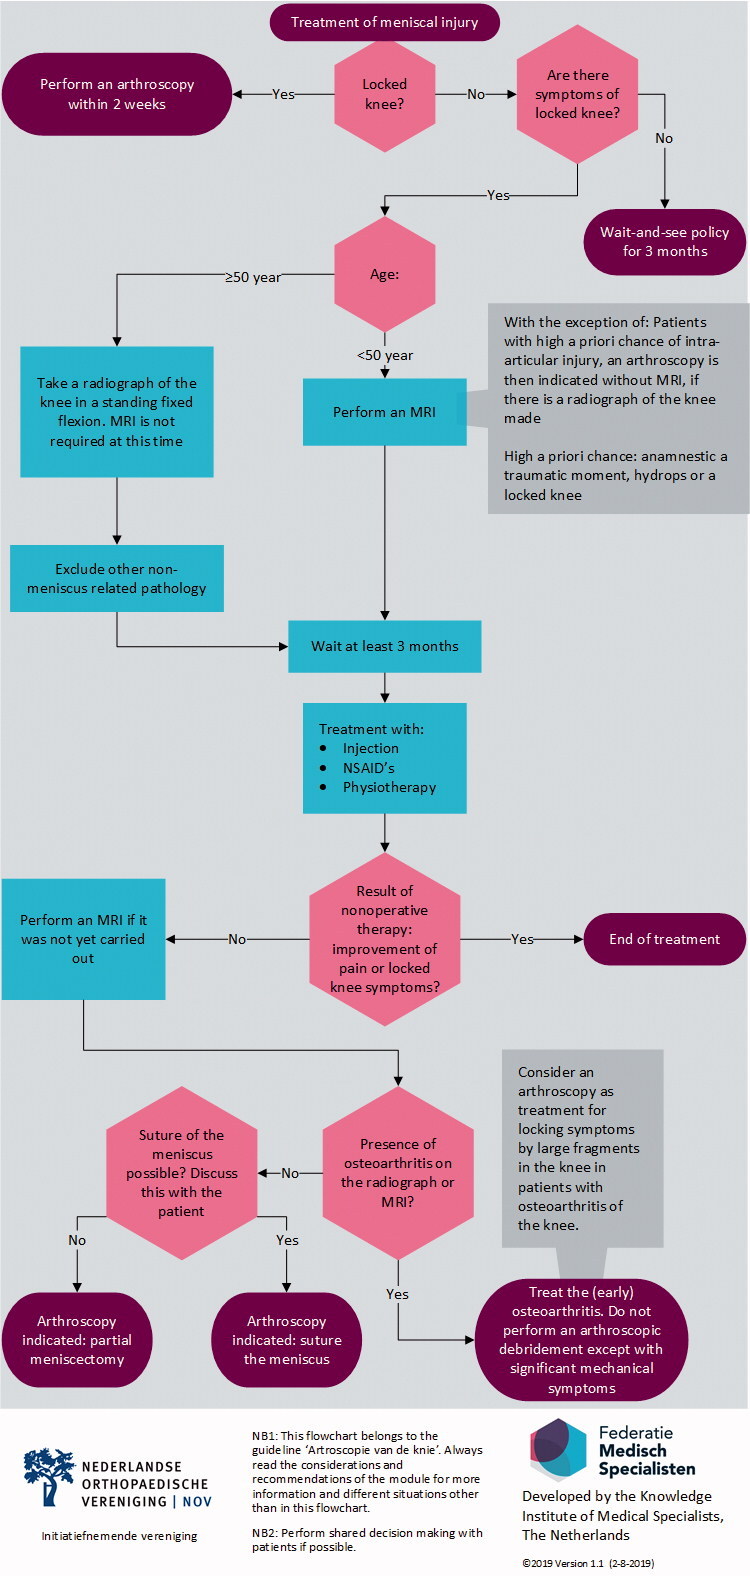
The 2nd question concerned the place and reliability of imaging of ultrasound compared with MRI techniques in the diagnostic process. Prior to arthroscopy osseous pathology (fracture, neoplasm, and infectious processes such as osteomyelitis) should be excluded by imaging. A general MRI scanning protocol, suitable for the assessment of menisci, ligaments, and cartilage, should consist of scans in different planes: sagittal, coronal, and, where relevant, axial. In the choice of 35 sequences at least a combination of short TE (T1- or PD-weighted images) and T2-weighted images with or without fat suppression should be used. Because there is a learning curve in reading of MR images, experience and training of the reader will increase the accuracy of MRI (White et al. 1997). Experience of the reader has more influence on accuracy of MRI than field strength of the system (Krampla et al. 2009). Therefore, reading of the MRI by a (musculoskeletal) radiologist, with adequate training and experience, is essential to achieve the highest accuracy.
